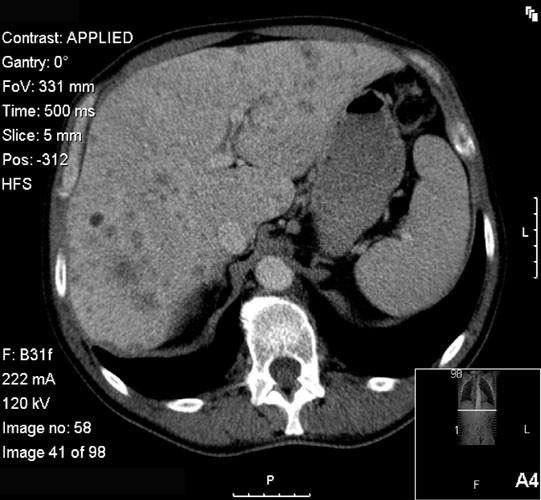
Thoracoabdominal-pelvic computed tomography scan showing several infracentimetric lymph nodes in the hepatic hilum.
